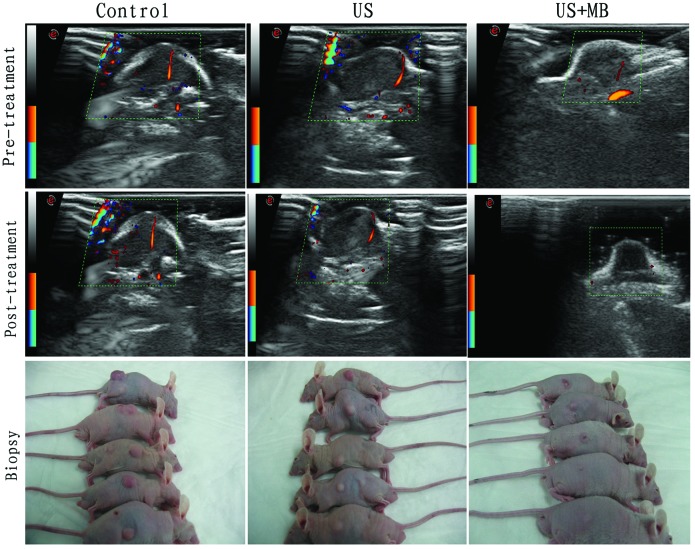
CDFI prior to and following treatment in the control, US and the US+MB group. Following 2 weeks of treatment, the blood flow signal in the US+MB group disappeared, and the tumor size was smaller when compared with the other 2 groups. CDFI, color Doppler flow imaging; US, ultrasound; MB, microbubble.
